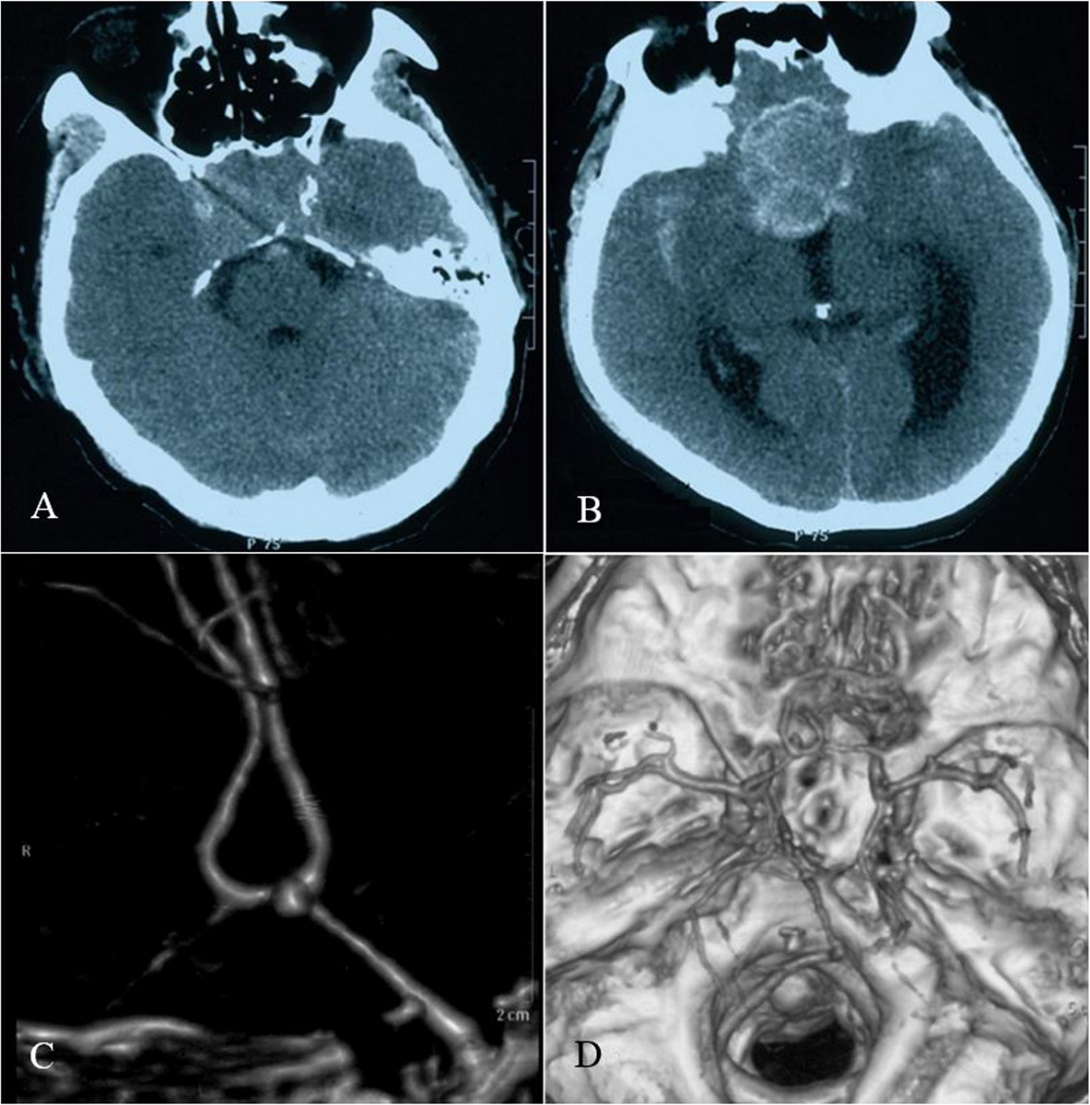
Cranial CT and CTA images. a, b Cranial CT showed space-occupying lesions in the sellar region and local bone destruction. At higher levels, the tumor image could be observed, and the boundary was clear. There appeared to be a capsule, the size of which was approximately 3 cm × 4 cm. The density inside and surrounding the tumor increased, patchy hemorrhage was observed, and a cord-like high-density image was observed in the bilateral sylvian cisterns. The findings were considered to be consistent with subarachnoid hemorrhage. c, d Cranial CTA revealed a cystic-like protuberance, identified as an aneurysm, in the anterior communicating artery. The neck of the aneurysm shifted toward the A1 segment of the left anterior cerebral artery. The left anterior cerebral artery was thicker. The Willis Circle was compressed and pushed to the lateral side
